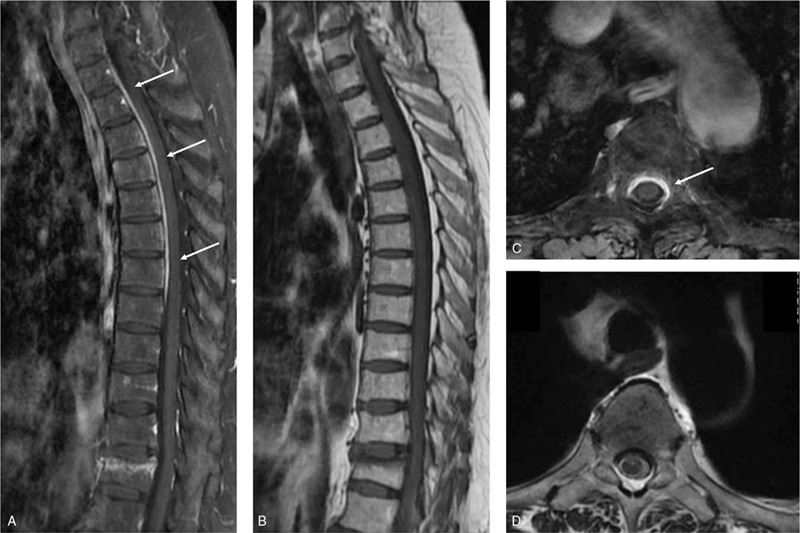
Contrast-enhanced MRI findings. (A) Sagittal MRI (T1WI+contrast-enhanced (CE)) of the spine showed diffuse thickening and enhancement of the frontal portion of the dura mater at upper to middle thoracic cord. (B) No obvious abnormality was noted on T2WI. (C,D) Axial MRI (C, T1WI+CE; D, T2WI) at the level of T4 showed changes at corresponding regions. Arrows: enhancement of the thoracic spinal dura mater.
